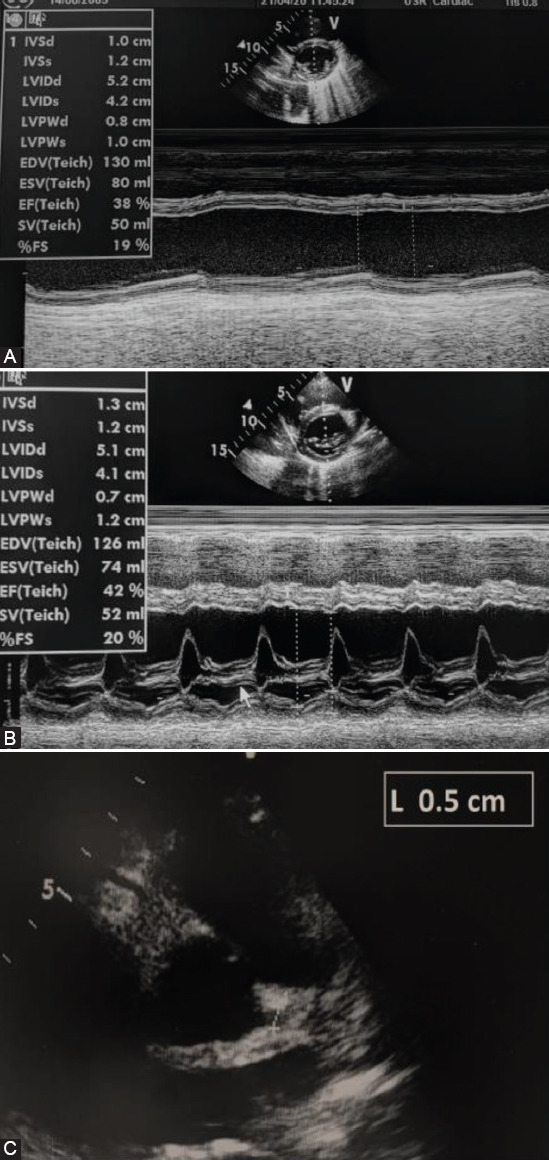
Echocardiography examination at the admission of our patients. (A) 1° patient: Left ventricular function – short axis; normal left ventricular dimensions with an increased wall thickness and decreased ejection fraction of the left ventricle. (B) 2° patient: Left ventricular function – short axis; normal left ventricular and decreased ejection fraction of the left ventricle. (C) 2° patient: Diameter of the left coronary artery (5 mm; Z score +2.77).
